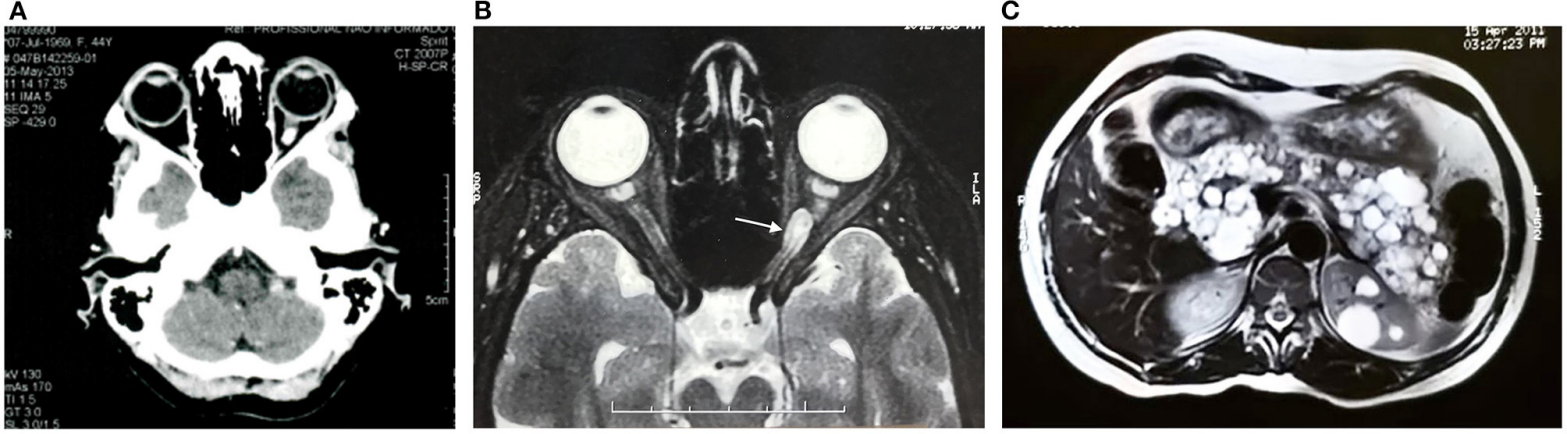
Computed tomography (A) and magnetic resonance imaging of the skull and orbit (B), and computed tomography of the abdomen (C) of the von Hippel- Lindau syndrome proband. (A) Left optic nerve thickening with tortuosity and an enhancement area with nodular aspect. (B) Left intraorbital tumor on T1-weighted imaging (white arrow). (C) Pancreatic cysts and renal cysts.
